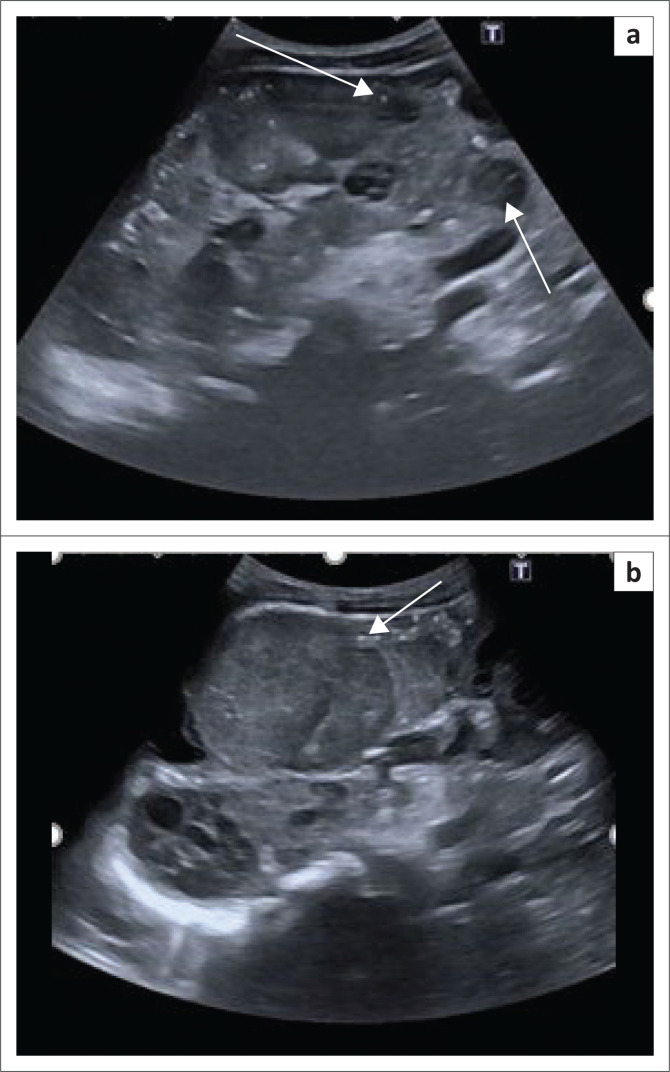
(a) Eleven-year-old male. Longitudinal greyscale ultrasound right kidney. Multiple markedly hypoechoic parenchymal masses were demonstrated (white arrows). (b) Longitudinal greyscale ultrasound left kidney. Large midpole mildly hypoechoic mass (white arrow).
